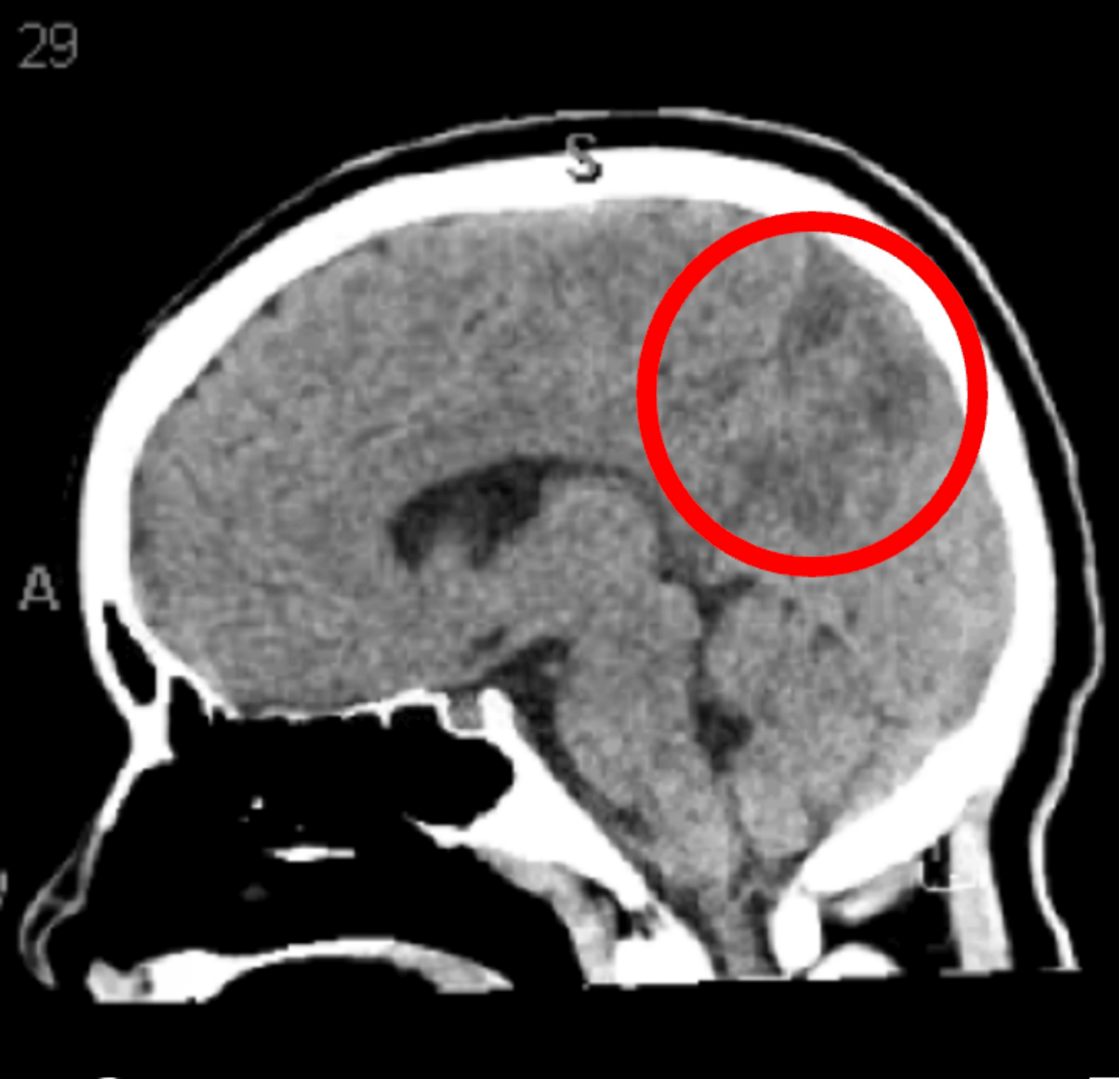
Sagittal non-contrast computed tomography brain scan The red circle represents hypodensity in the right posterior parietal lobe, suggesting posterior reversible encephalopathy syndrome.
